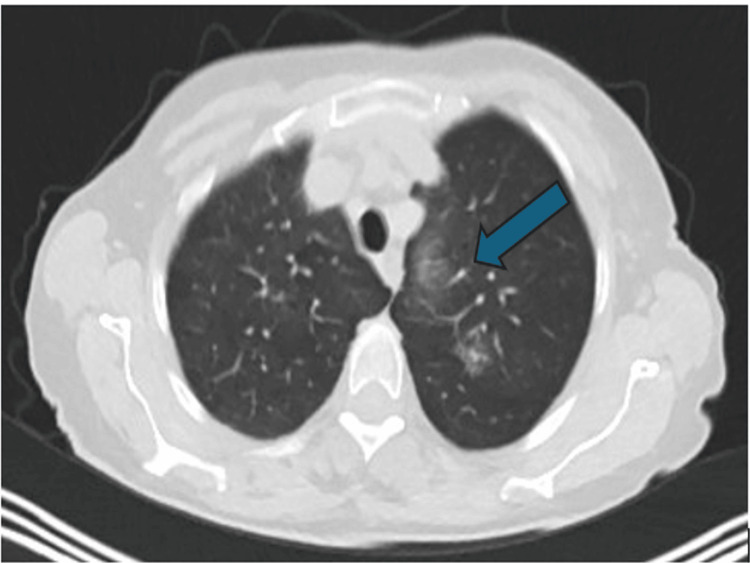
CT scan showing right lower lobe lesion
